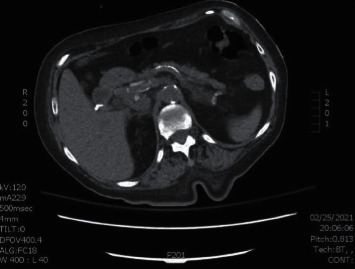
CT scan image of the abdomen without contrast showing resolution of the pancreatic mass after allopurinol therapy.
